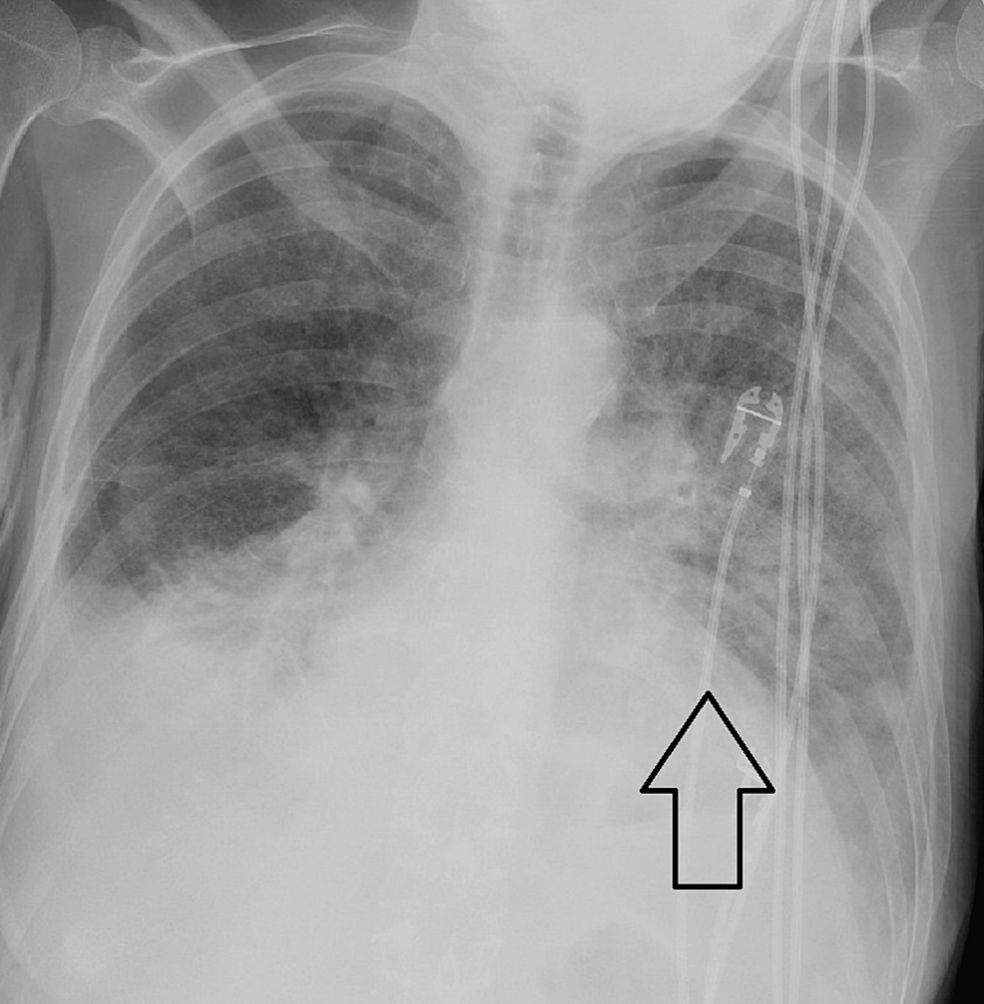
Chest x-ray immediately after hyperbaric oxygen session demonstrating acute pulmonary edema with prominence in the left lung (arrow)
